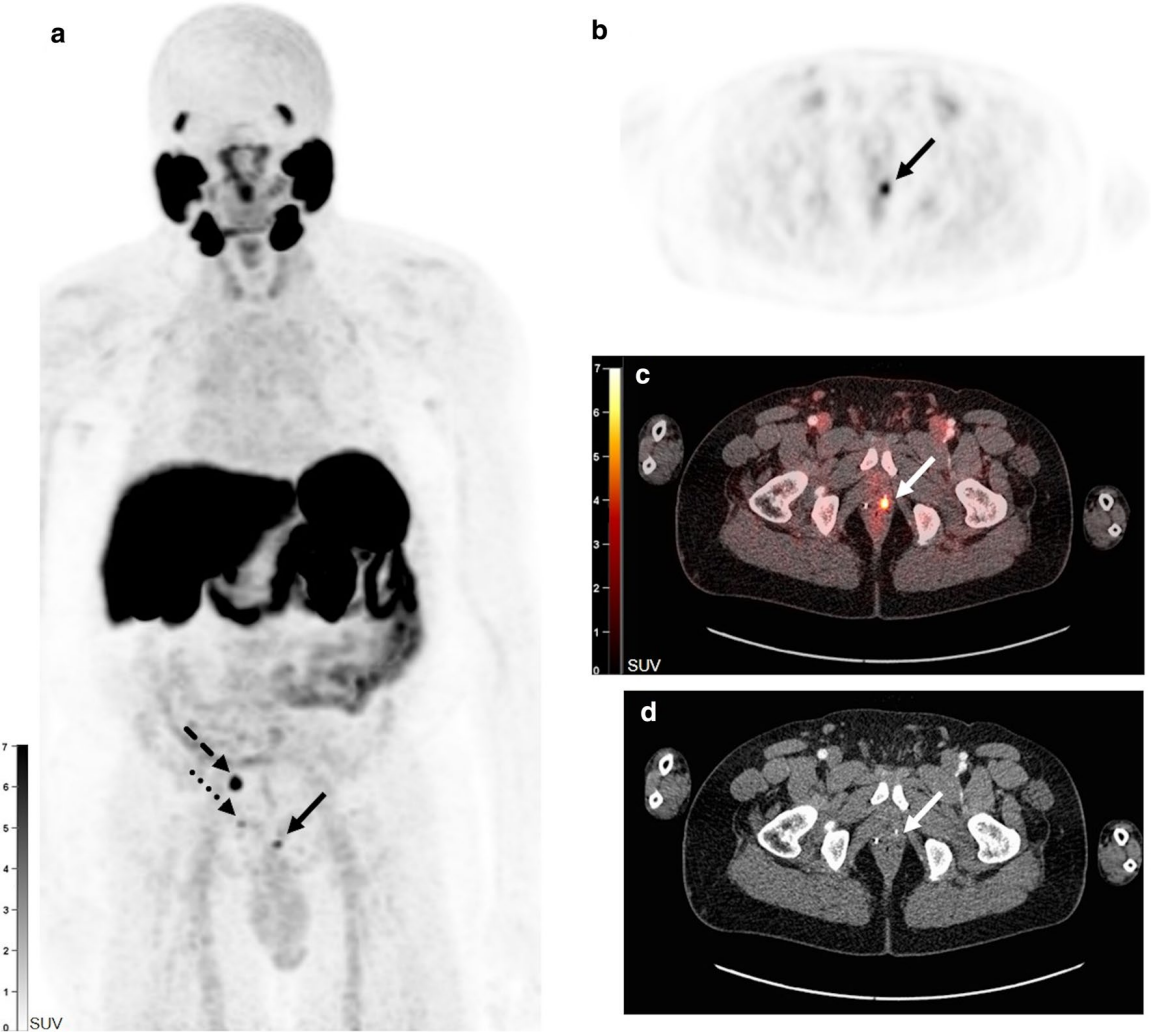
[^18^F]PSMA-1007-PET/CT scan (MIP image (a) and transversal PET (b), fusion (c) and CT images (d)) in a 64-year old patient with a medical history of radical prostatectomy with extended lymphadenectomy (Gleason score 9, pT3bN0). PSA value at the moment of [^18^F]PSMA-1007-PET/CT scan was 2.27 ng/ml. A local recurrence was detected in the prostatectomy fossa on the left side (a–d, full arrow) and in the right seminal vesicle (a, dotted arrow). Also, a malignant lymph node was visible at the right internal iliac artery (a, dashed arrow). The patient was treated with salvage external beam radiation therapy and androgen deprivation therapy with subsequent decline of PSA to 0.01 ng/ml 7 months later
